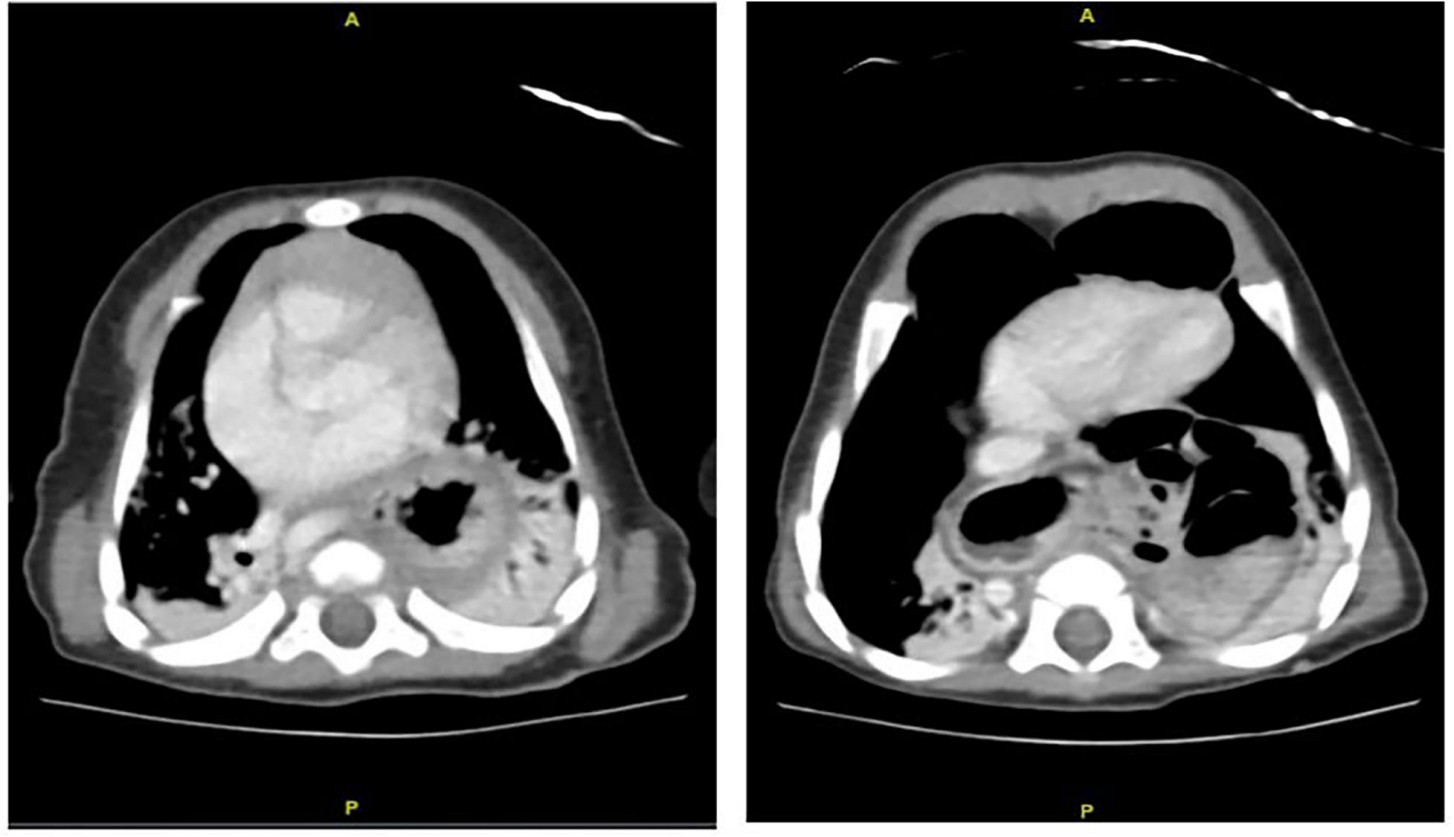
CT scan of the chest and abdomen of the patient shows a paraesophageal hernia with an approximately 3.5 cm defect, associated with superior herniation of the stomach and bowel loops. Another defect is noted at the diaphragm anterior to the heart, measuring approximately 3.22 cm, associated with herniation of the transverse colon into the anterior mediastinum, representing a Morgagni hernia. There is a bilateral consolidation and atelectasis within the lower lobes.
